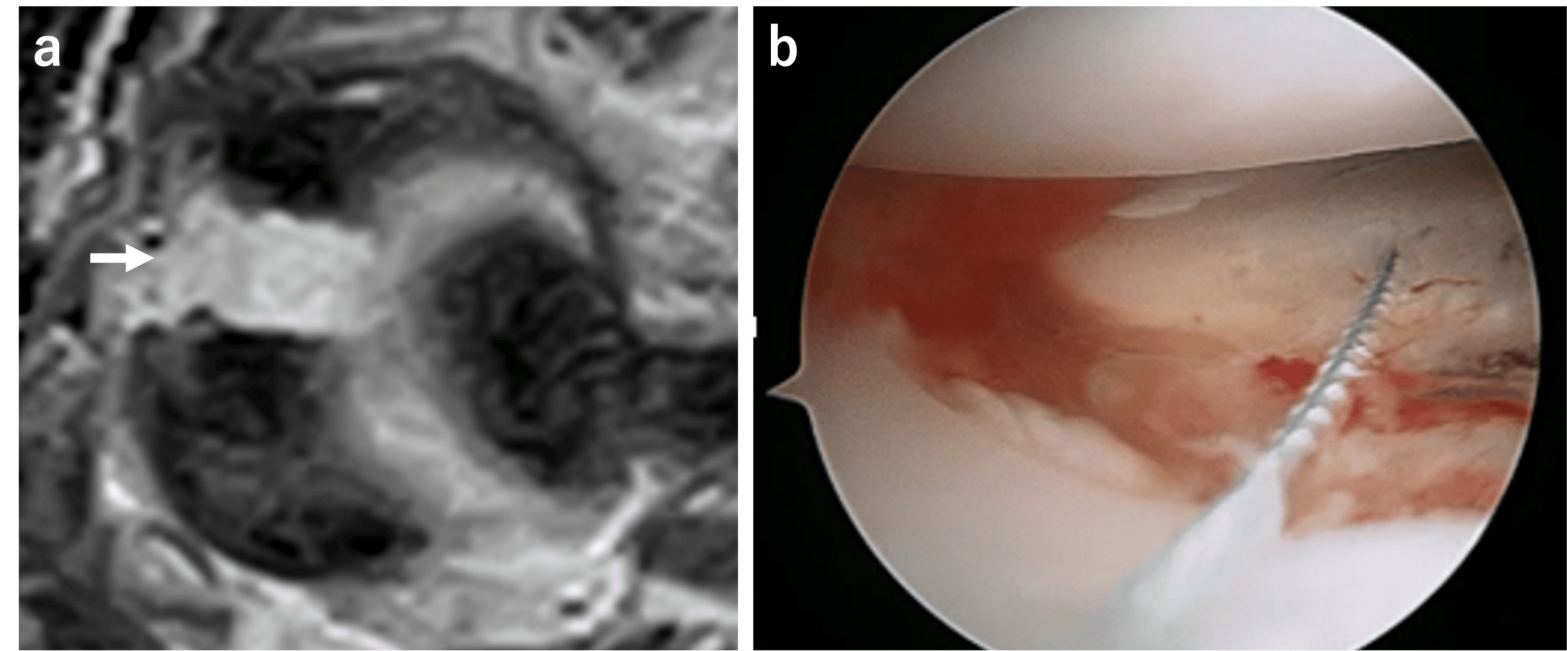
Failure of the repaired meniscus (a) Axial view on MRI showed re-diastasis of the repaired site (white arrow) (b) Rearthroscopy showed the broken repair site with the cutout of suture from the meniscus
